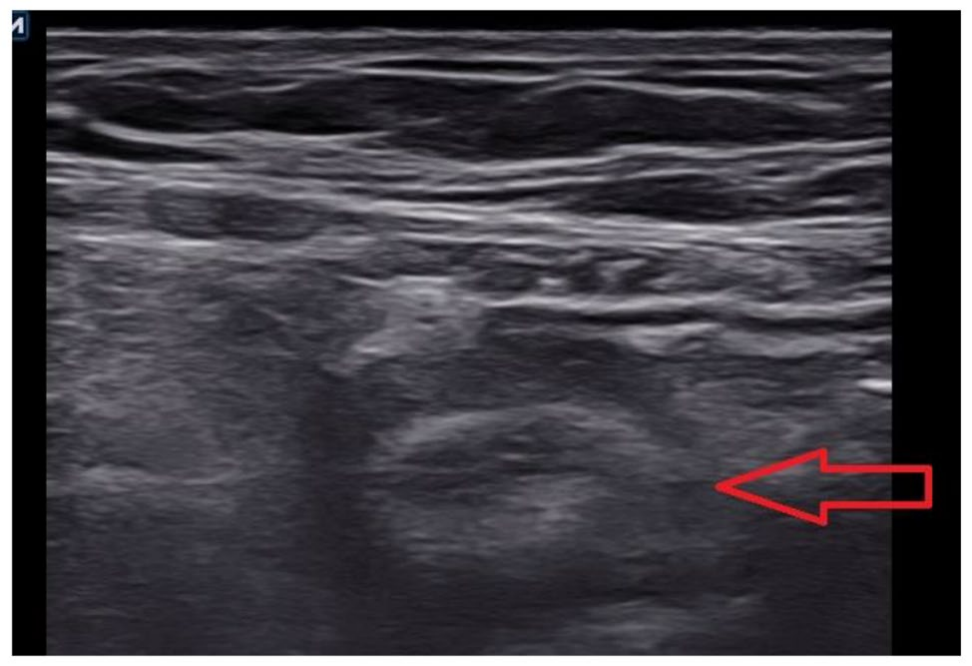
Round, aperistaltic, noncompressible and dilated appendix; distinct appendiceal wall layers; periappendiceal fluid collection; and wall thickening on sonography
